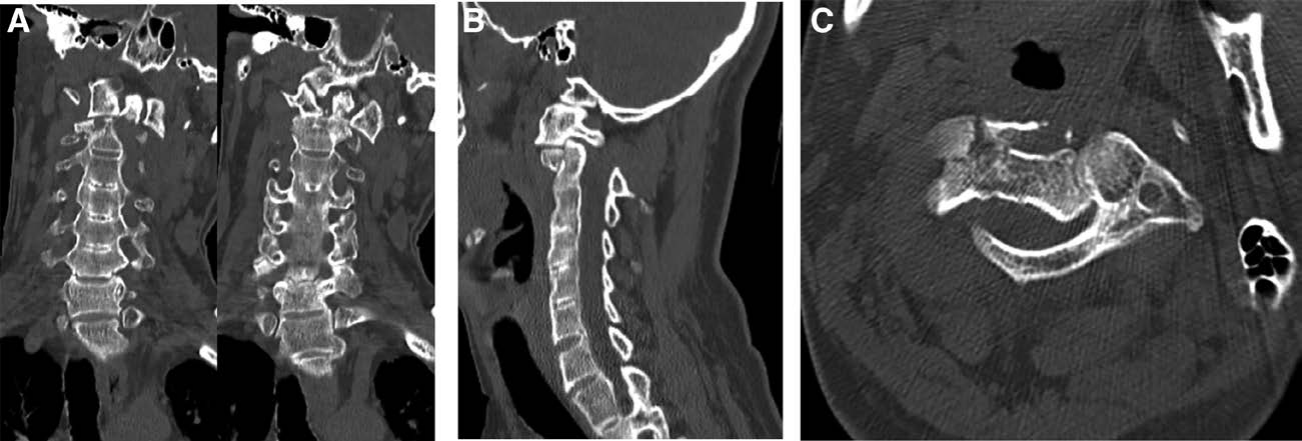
Preoperative coronal, sagittal and axial images. A coronal CT scan also revealed left lateral dislocation of C1 on C2 with a horizontal translation of the odontoid tip (A). On the axial and sagittal CT scan, the left C1 lateral mass was displaced anteriorly and locked by the C2 body (B and C). CT = computed tomography.
